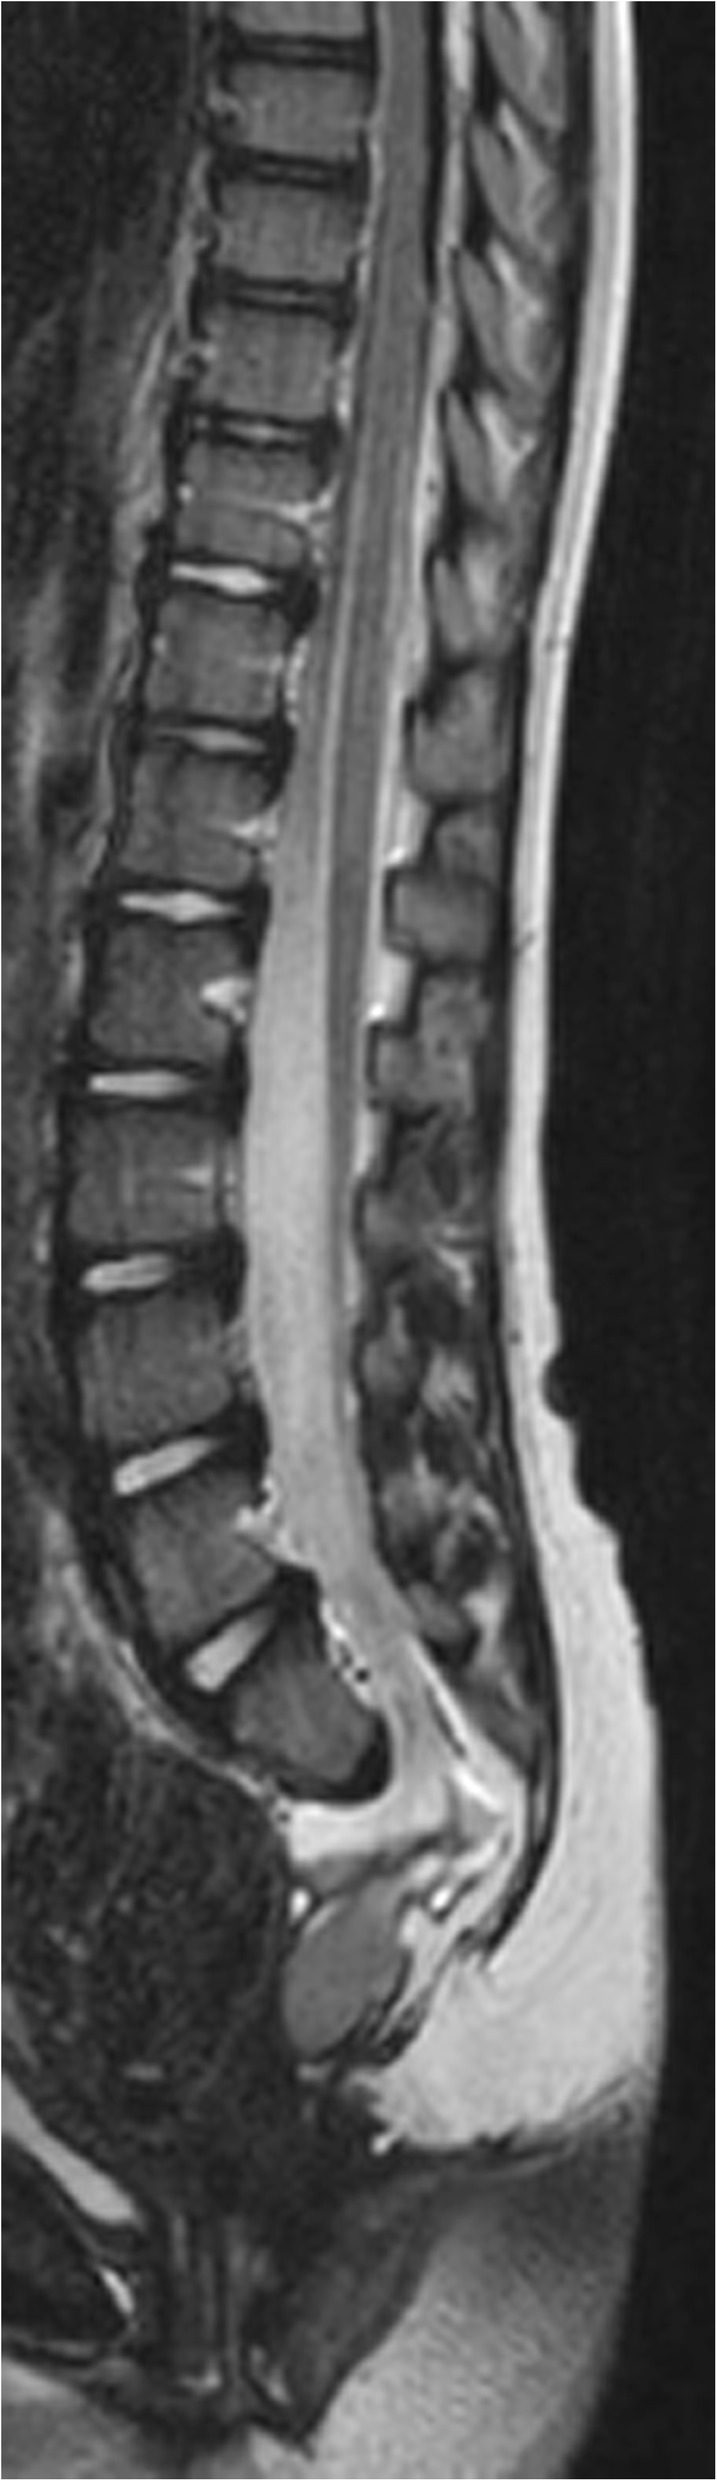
Sagittal t2- weighted Lumbosacral MRI
